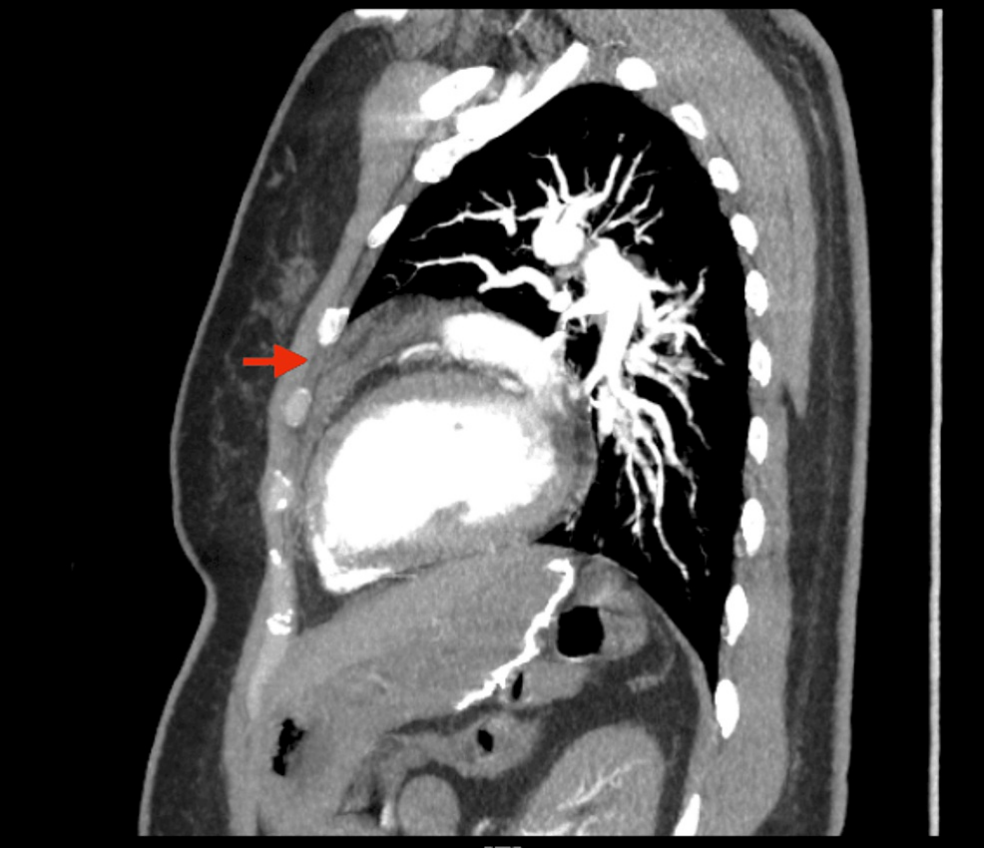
Sagittal view CT chest. Red arrow indicating the anterior hematoma within the mediastinum.
